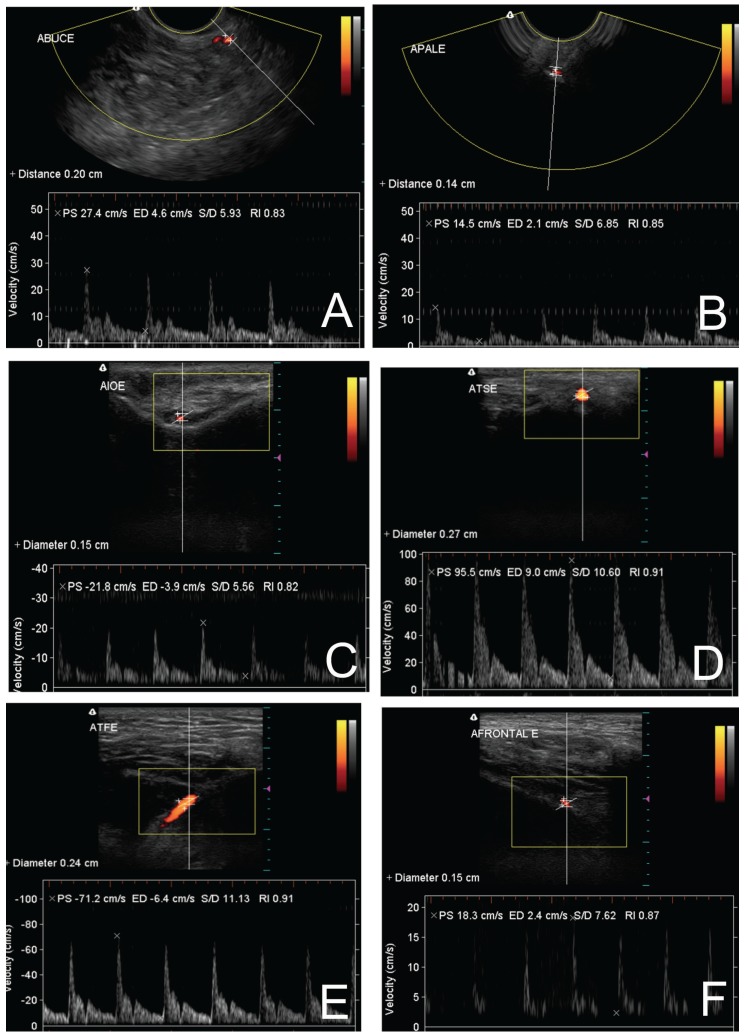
Ultrasonographies of the intraoral arteries: A- deep lingual a., B- sublingual a., C- greater palatine a., D- inferior alveolar a., E- bucal a., F- mental a.
